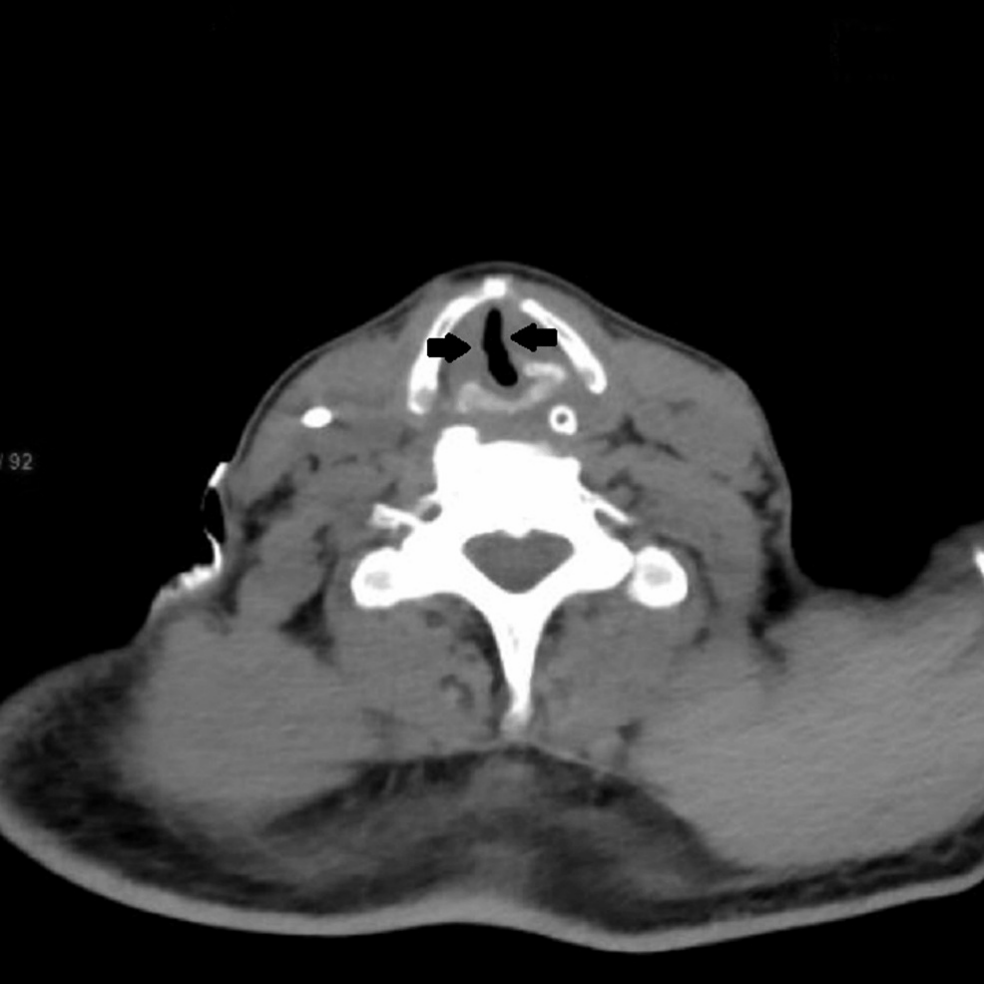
CT of the neck showing soft tissue edema with significant airway compromise
